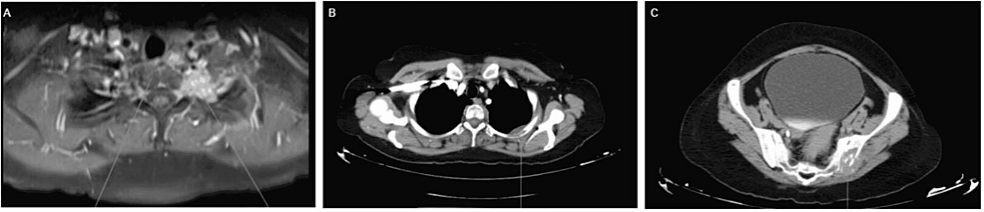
MRI and CT of metastatic lesions A: MRI of the hip - in the lower margin of the left interscalene triangle, a poorly defined mass can be seen that compromises the T1 and T2 roots;  B: CT of  the thorax - osteolytic lesion seen on the posterior aspect of the left third costal arch associated with an ill-defined oval soft tissue density mass of approximately 28 x 17 mm;  C: CT of abdomen and pelvis -  ill-defined osteolytic lesion seen in the left iliac flap with a soft tissue component of approximately 47 x 31 mm
